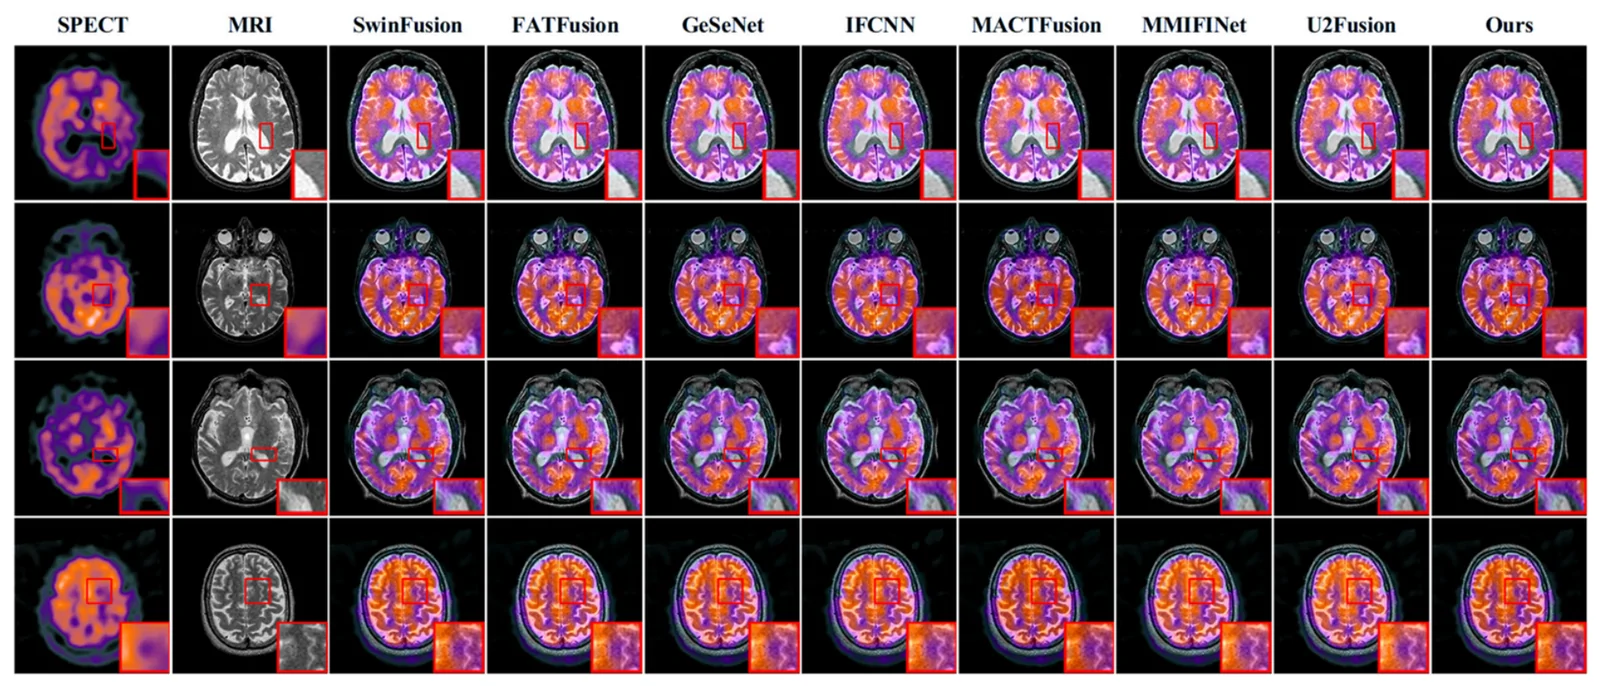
Qualitative comparison of fusion results on the SPECT–MRI dataset. Given the low-contrast and noise-prone nature of SPECT images, this comparison focuses on the preservation of functional activity regions against the anatomical background. MSFusion demonstrates superior capability in suppressing background noise while clearly delineating the functional uptake regions, avoiding the over-smoothing or severe artifact introduction seen in other methods.
